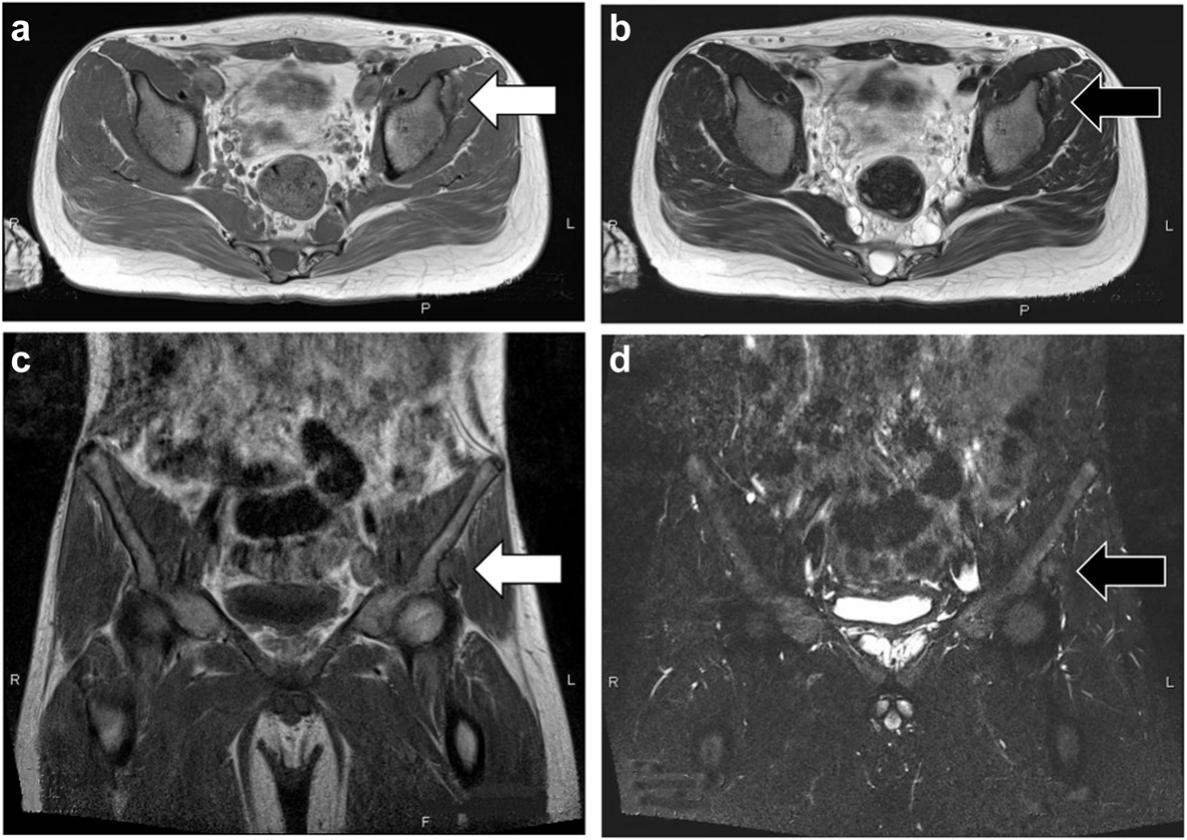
Axial MRI showed a soft tissue mass adjacent to the anterior inferior iliac spine, which was isointense on T1WI (*white arrow*) (a) and T2WI (*black arrow*) (b). Coronal images showed isointensity in T1WI (*white arrow*) (c) and T2WI with fat saturation image (*black arrow*) (d)
